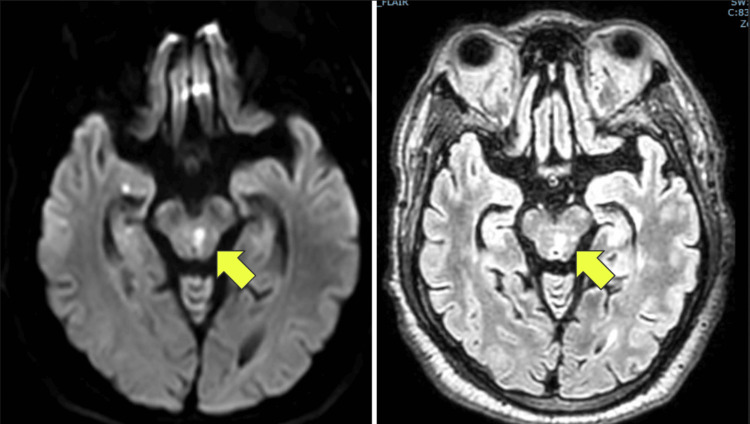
MRI brain demonstrating a lacunar infarction in the left dorsal superior pons extending up into the midbrain (yellow arrows)
